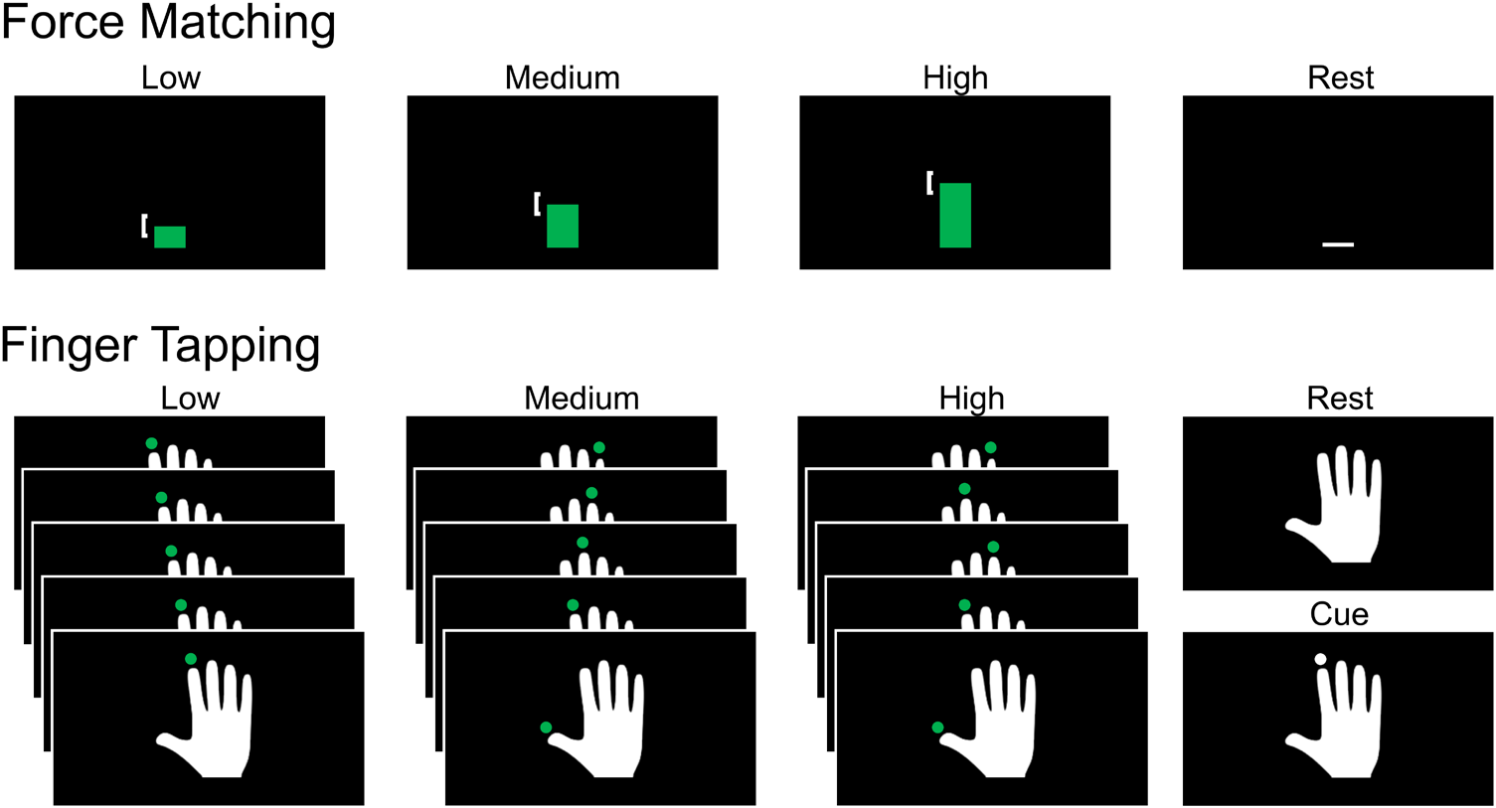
Visual cues for the force matching and finger tapping tasks. For the force matching task, participants continuously gripped an MRI-compatible dynamometer with their right hand to match 10%, 20%, and 30% of their maximum voluntary contraction, corresponding to low, medium, and high task levels, respectively. Visual cues were provided with a vertical white bar and a white square bracket that spanned the target force ±1 kgf. The white bar turned green when the force applied to the dynamometer was within the square bracket. For the finger tapping task, participants responded by pressing buttons on an MRI-compatible five button response pad with their right hand. Visual cues were provided by placing a white circle at the distal aspect of the digits of a white hand, indicating when and which button to press. The circles appeared at a rate of 1 Hz, remained visible for 900 ms, and turned green if the correct button was pressed. The experiment had three task levels: a single-digit response with the second digit only, a single-digit response with all digits in a sequential order, and a single-digit response with all digits in a random order, corresponding to low, medium, and high task levels, respectively.
